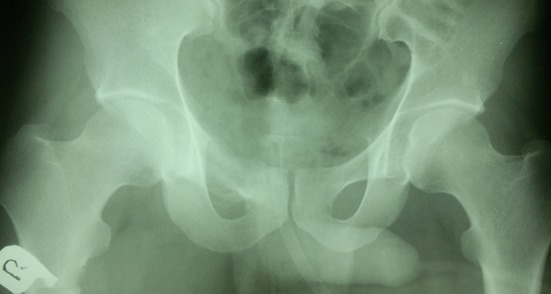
Postoperative radiograph of pelvis showing complete excision of the bony growth.
